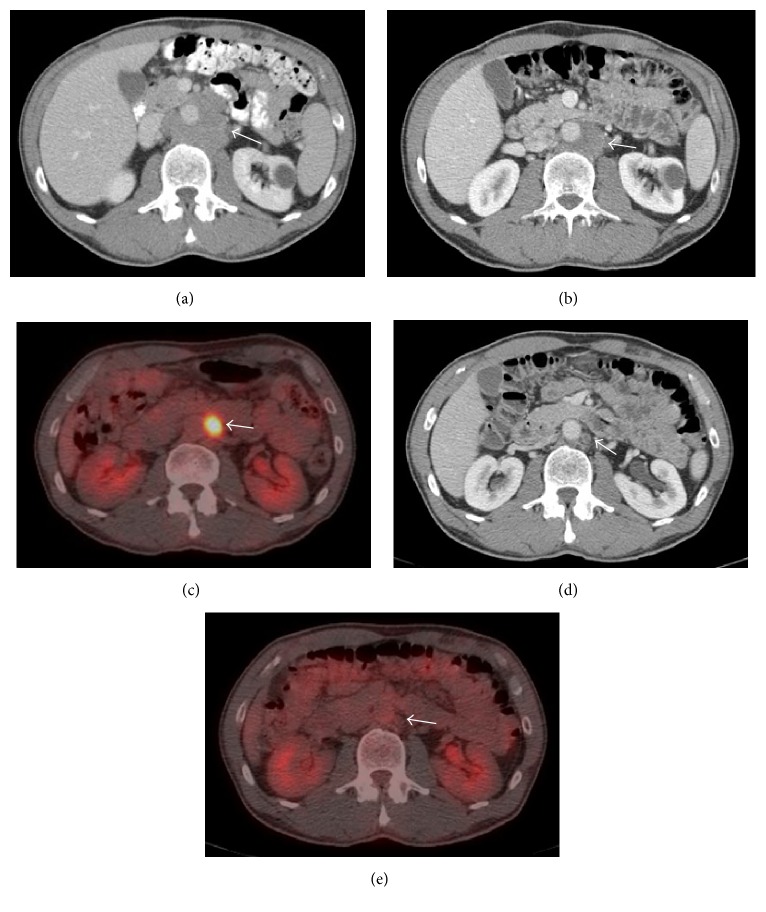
Representative images from serial computed tomography (CT) and ^18^F-fluorodeoxyglucose- (FDG-) positron emission tomography (PET) scans showing resolution of the biopsy-proven aggressive B-cell non-Hodgkin lymphoma in a conglomeration of periaortic lymph nodes. (a) CT image prior to diagnosis. (b and c) CT and FDG-PET images (resp.) at time of diagnosis showing interval decrease in size of the mass. (d and e) CT and FDG-PET images (resp.) six months after initial diagnosis, showing resolution of the mass. Arrows denote the location of the mass.
